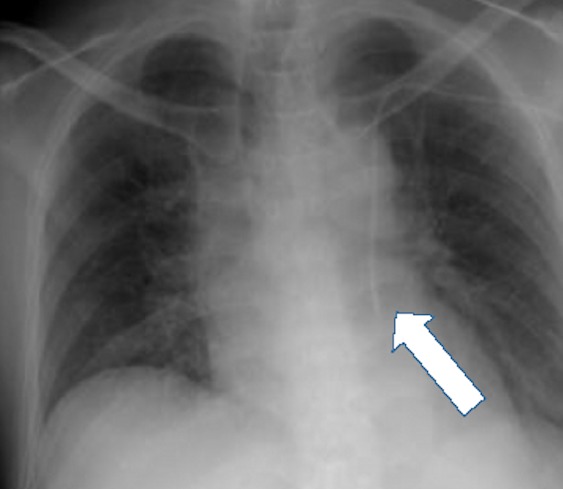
Portable chest radiograph showing a central line inserted in the left subclavian vein, catheter located at persistent left-sided superior vena cava (arrow). Patient was unaware of his congenital variant. He was always asymptomatic during line placement.
